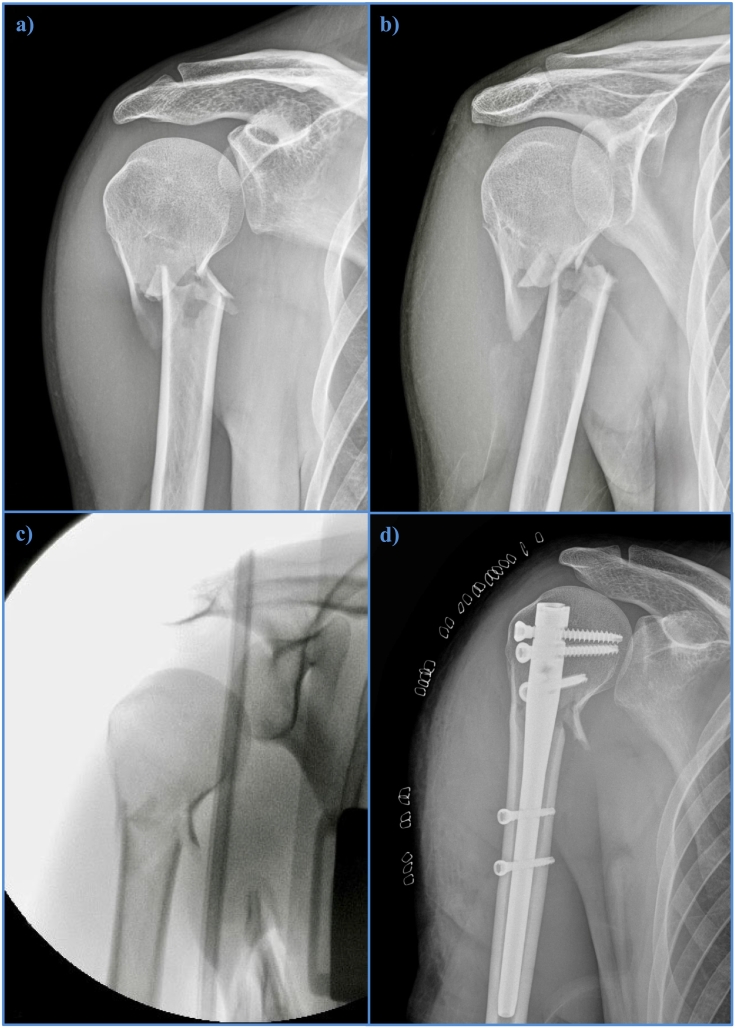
a-b) Fracture pattern c) after closed reduction d) post-operative X-rays.
